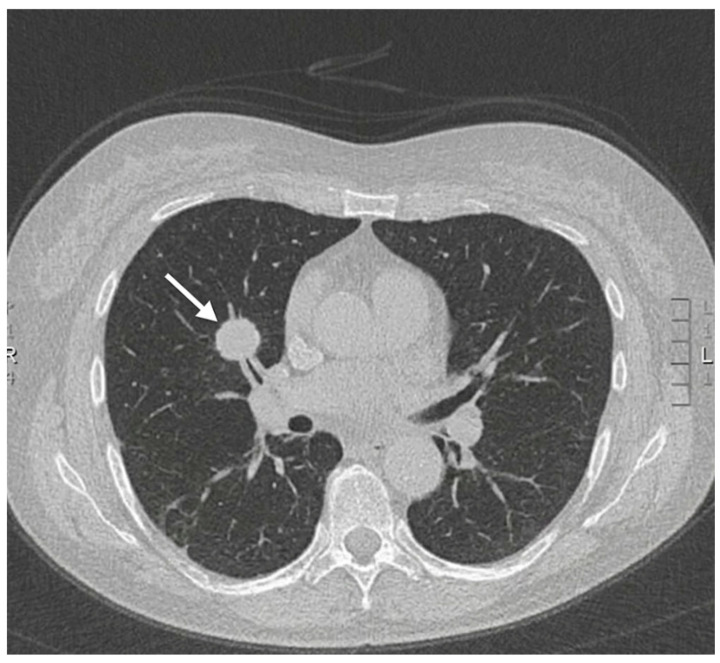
CT of the chest. A pulmonary nodule is noted in the right middle lobe (white arrow).
